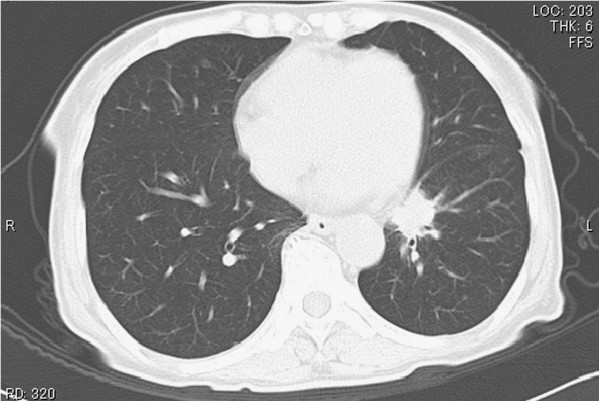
Chest computed tomography imaging on admission showed the primary lesion in the left lower lobe.
